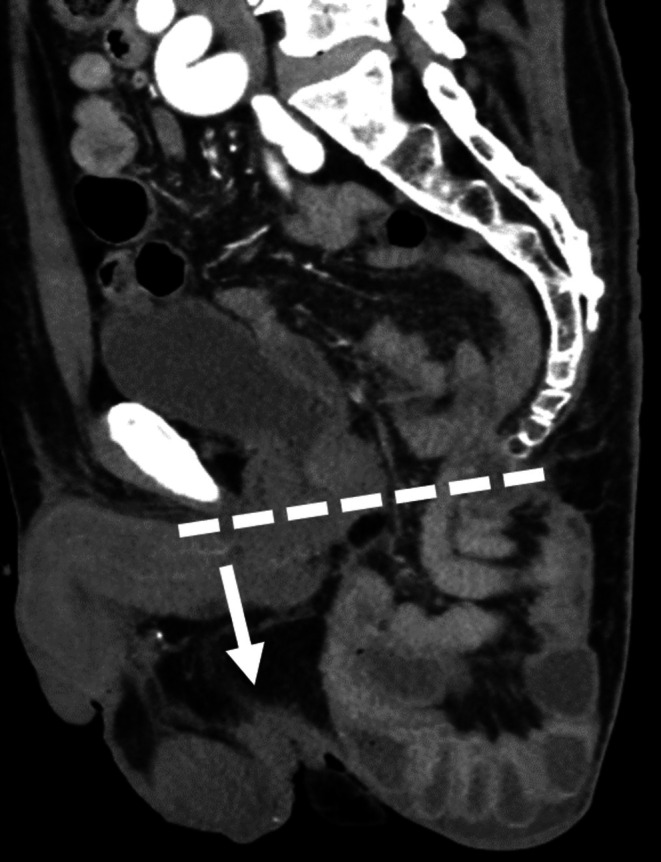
Definition of perineal hernia. Perineal hernia was defined as the downward displacement of the intestine beyond the line drawn by computed tomography from the inferior margin of the pubis to the edge of the coccyx, as previously reported.
